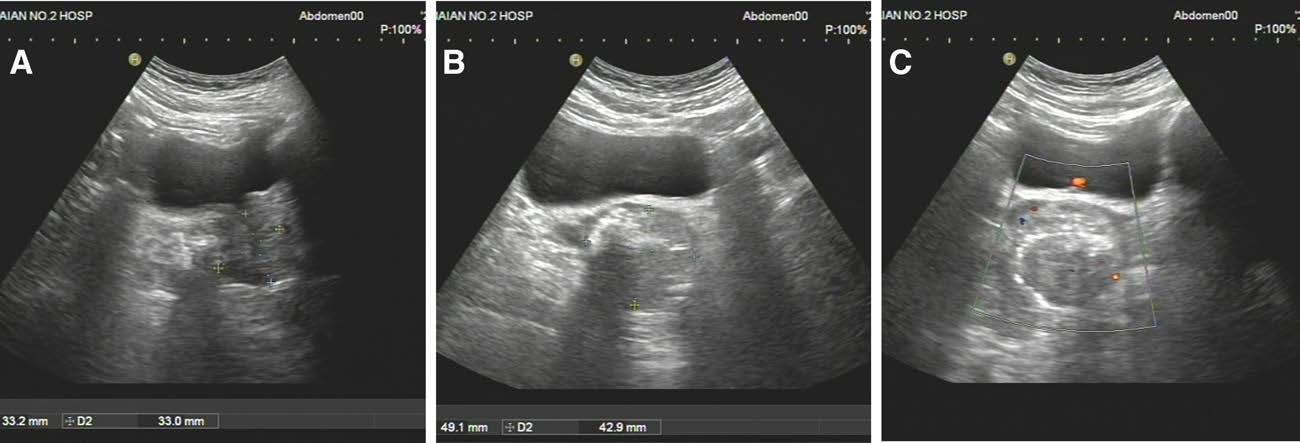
(A–C) Prostate ultrasound (US) showed an increase in prostate volume, with a mixed echogenic mass of approximately 4.9 × 4.3 cm in size visible on the left side of the prostate, most of which protrude outward from the contour, with irregular strong echoes visible inside, and local blood flow signals. The left seminal vesicle gland is compressed.
